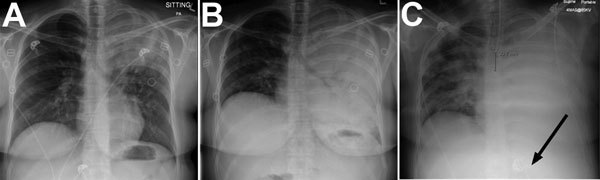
Chest radiograph at various stages of *Blastomyces gilchristii* infection in a 27-year-old woman, Ontario, Canada. A) Day 0: posterior–anterior (PA) chest radiograph at initial emergency department examination. Discrete confluent left upper lobe consolidation with air bronchograms are visible. B) Day 5, 15:10: PA chest radiograph demonstrating complete confluent opacification of the left hemithorax with extensive air bronchograms. C) Day 6, 23:30: PA chest radiograph postintubation with confluent left lung consolidation and new right patchy airspace opacification. Arrow indicates the correct placement of a nasogastric tube.
